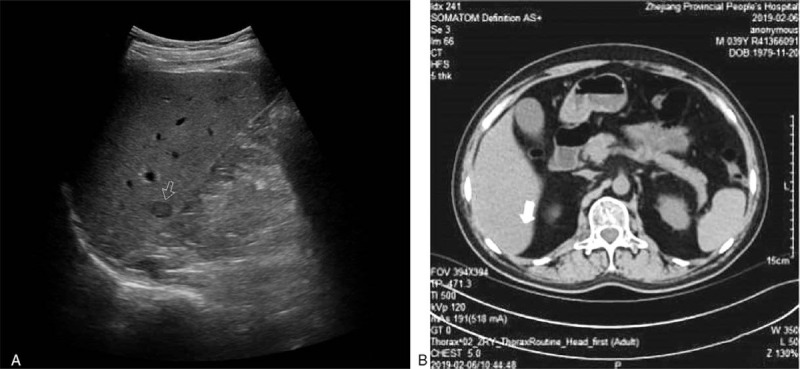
The same level of ultrasound and computed tomography (CT) images. (A) Ultrasound revealed a 1.5 cm × 0.9 cm hypoechoic nodule. (B) At the same level, computed tomography (CT) did not detect any solid lesion.
